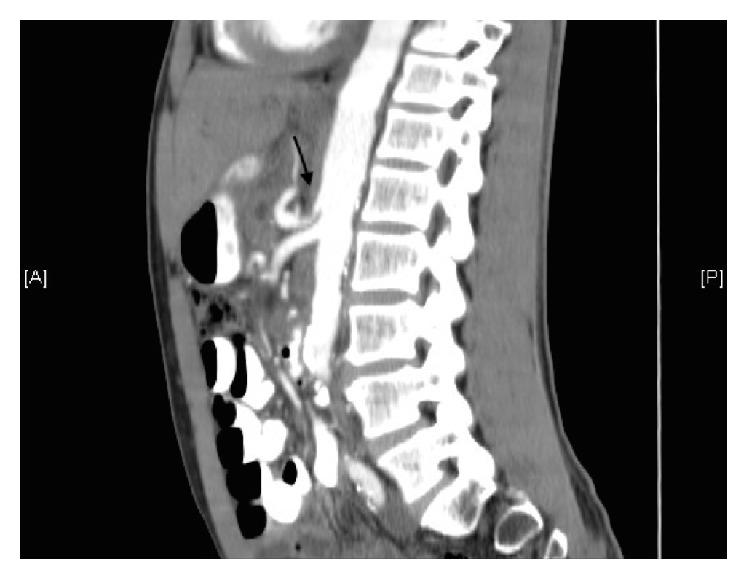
CT abdomen and pelvis sagittal view demonstrating severe stenosis at the origin of the celiac artery.
